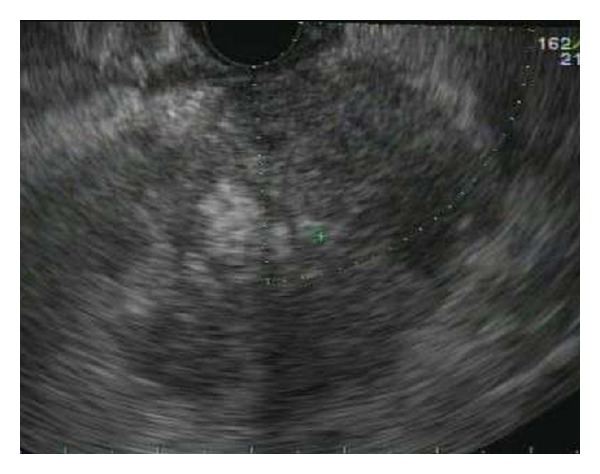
Endoscopic ultrasound image of a tumor in the head of the pancreas prior to intratumoral injection.
